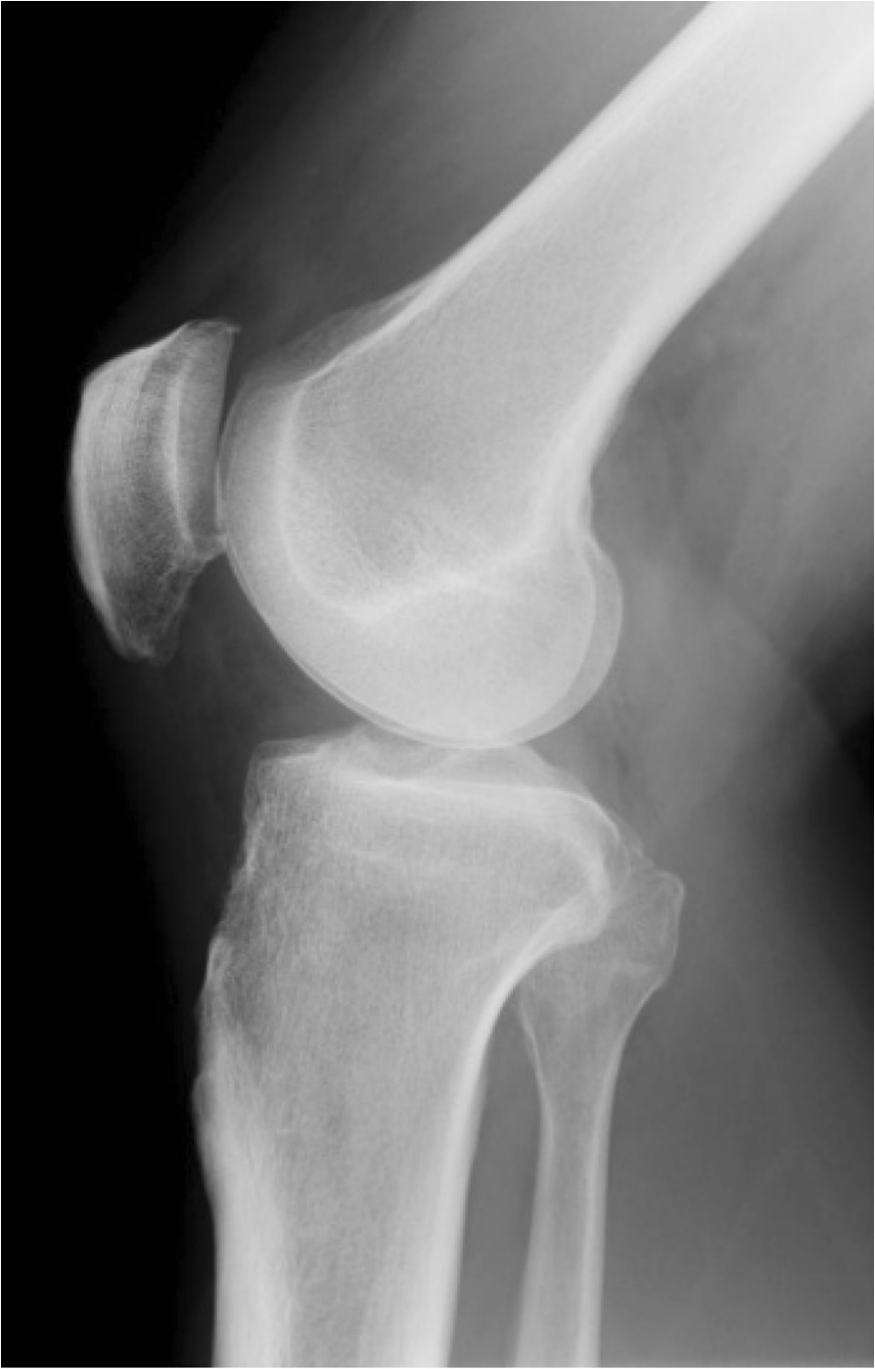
Radiographs 2 years after the operation. No signs of recurrence were observed.
